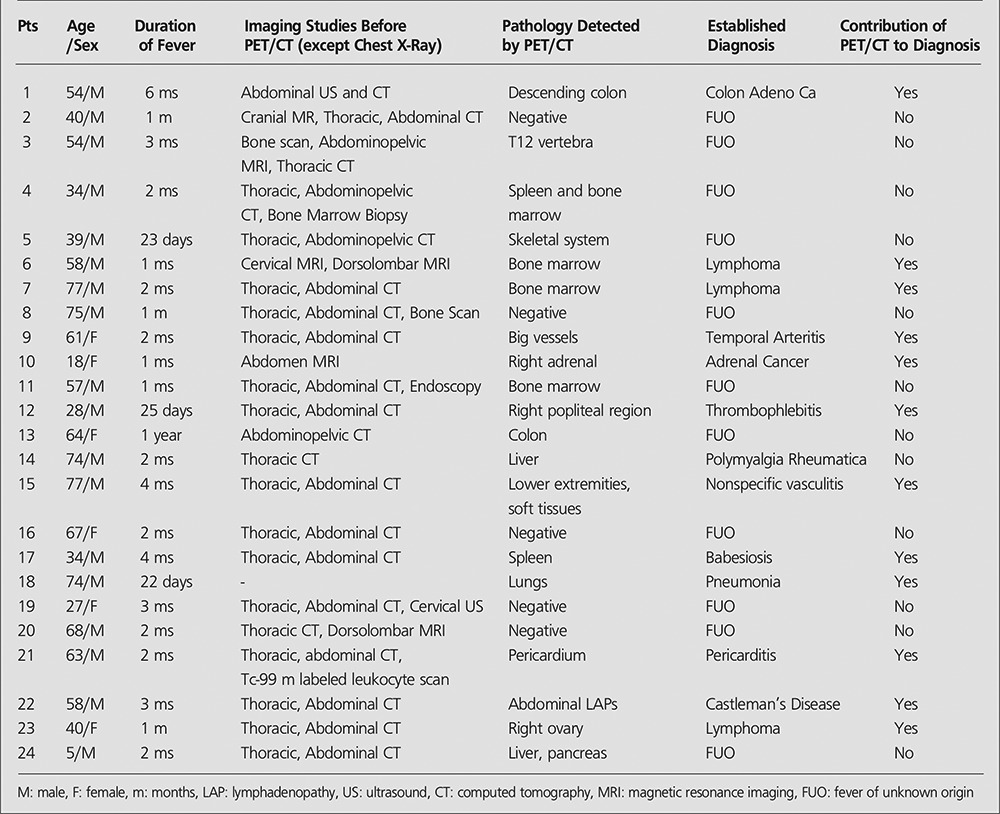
The patients’ characteristics, FDG-PET/CT results and final diagnosis
